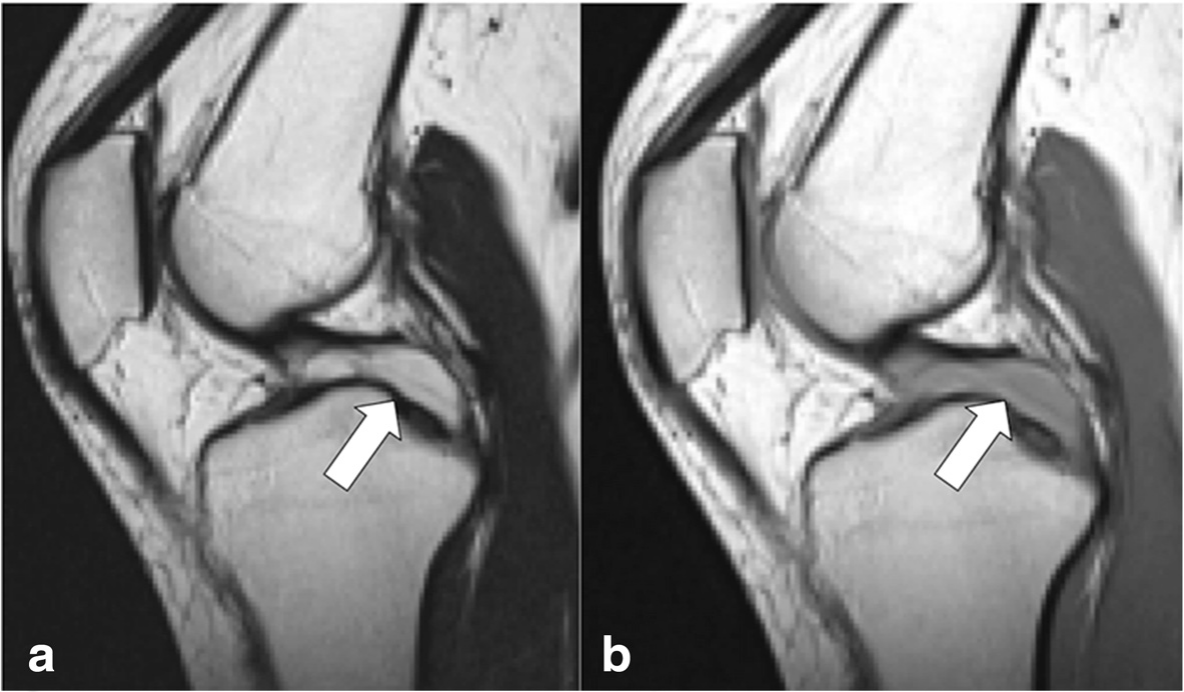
Sagittal magnetic resonance images revealed a cystic lesion located between the posterior cruciate ligament and intercondylar notch. This lesion exhibited apparent hyperintensity on T2 weighted images (T2WI, a) and slight hyperintensity on T1WI (b)
